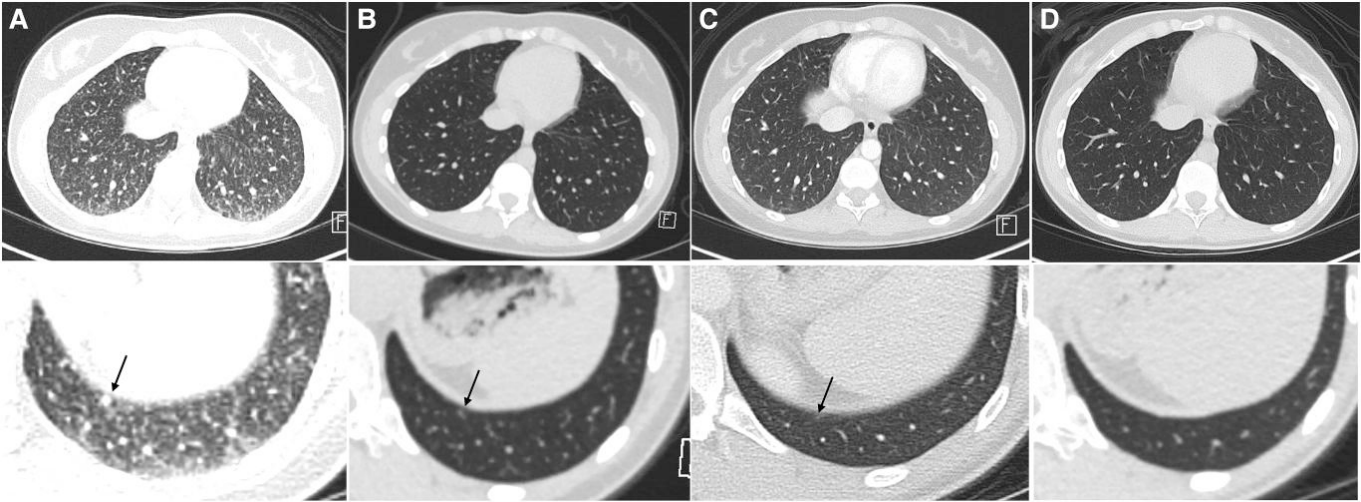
Computed tomography (CT) scan of the chest demonstrating changes in lung metastases after selpercatinib and radioactive iodine therapy. (A) CT scan of the chest with contrast before selpercatinib shows innumerable lung metastases (miliary pattern), with the dominant pulmonary nodule measuring 0.6 cm. The arrow points to one of the dominant subpleural solid nodules (0.6 cm) in the left lower lobe. (B) CT scan of the chest without contrast after 3 months of selpercatinib showed improvement/resolution of punctate pulmonary nodularity seen on the prior examination, consistent with favorable interval response to therapy. The arrow indicates a 3-mm nodule in the left lower lung. (C) CT scan of the chest with contrast 12 months after redifferentiation with selpercatinib and RAI 146 mCi (5402 MBq) shows a stable 3-mm subpleural pulmonary nodule. The arrow points to the 3-mm nodule in the left lower lung. (D) CT scan of the chest with contrast 24 months shows resolution of miliary pulmonary metastases.
